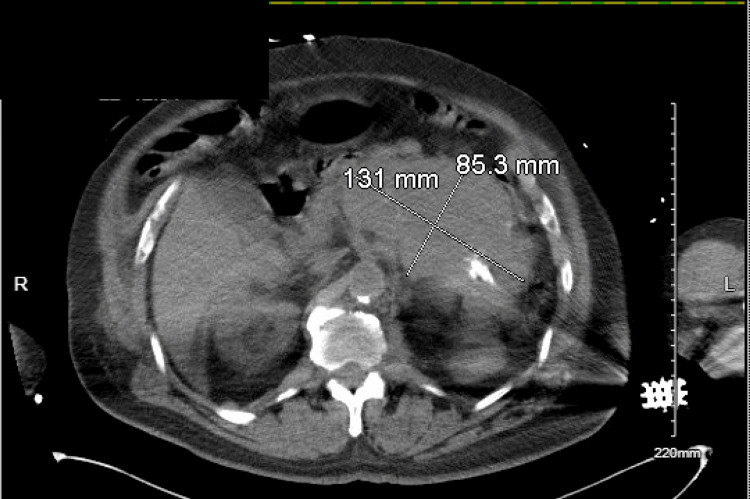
CT finding of a left paraaortic/retroperitoneal mass encasing the left side of the aorta, causing hydronephrosis of the left kidney CT: Computerized tomography
